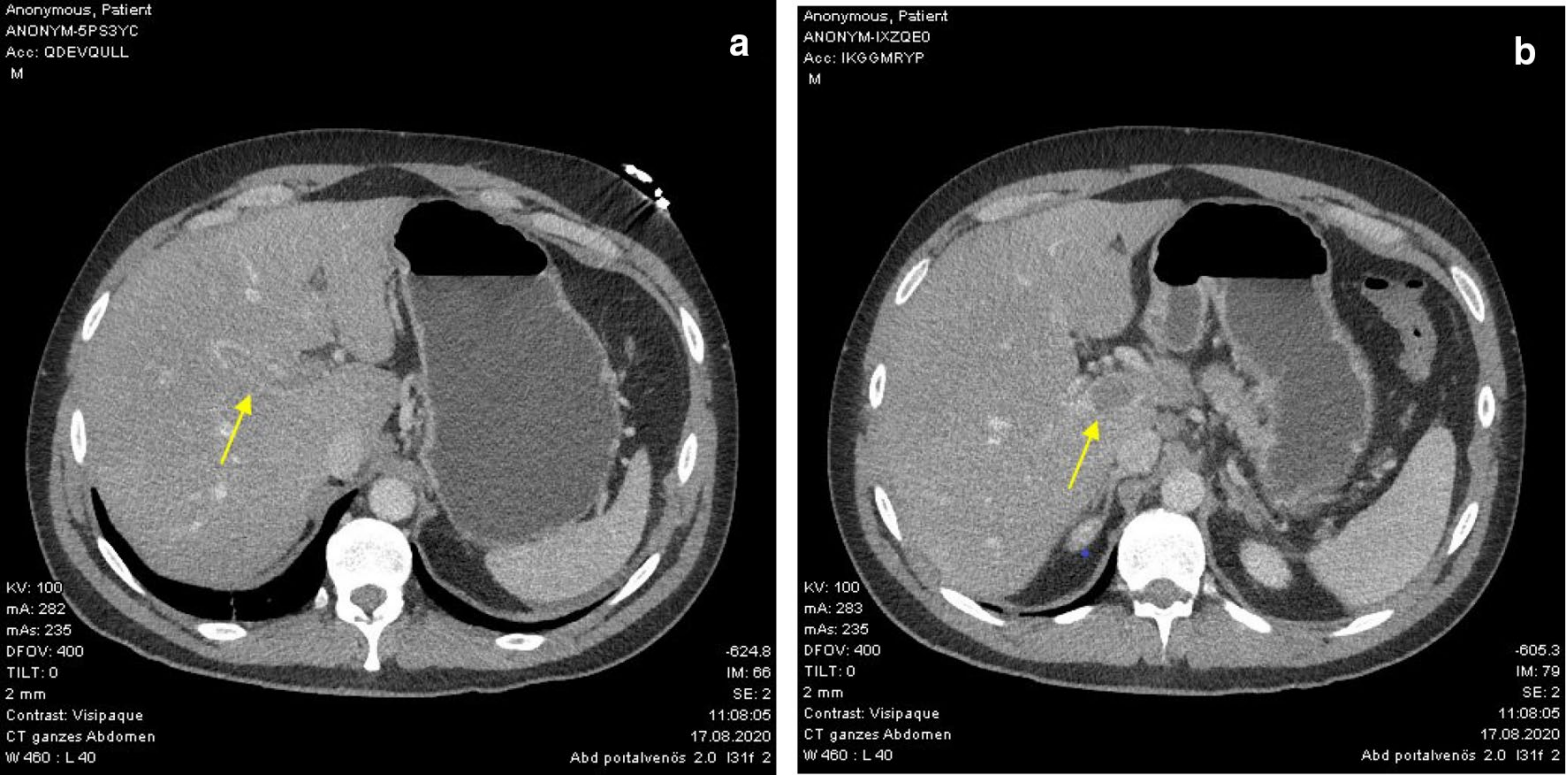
a, b CT-scan with portal vein thrombosis on admission, which occludes nearly the whole caliber (yellow arrows)
